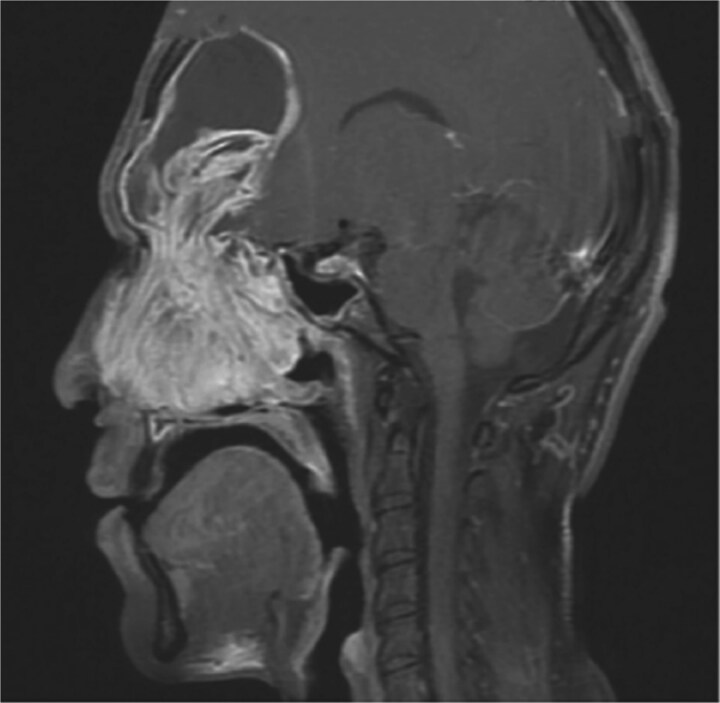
MRI sagittal view of the brain and sinuses showing mucopyoceles causing significant bilateral frontal sinus enlargement.
